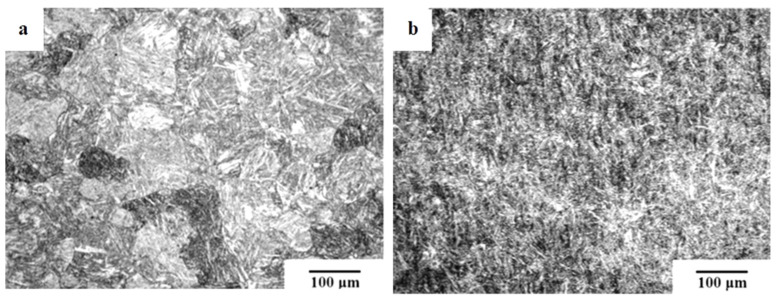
Optical microstructures of 0.15% C, 14% Cr, 12.5% Co, 4.5% Mo, and 2% Ni steel after different heat treatment processes. (a) Austenitisation at 1050 °C for 40 min, followed by oil quenching; (b) austenitisation at 1050 °C for 40 min, followed by oil quenching and CT at −196 °C for 2 h. Adapted from [70].
